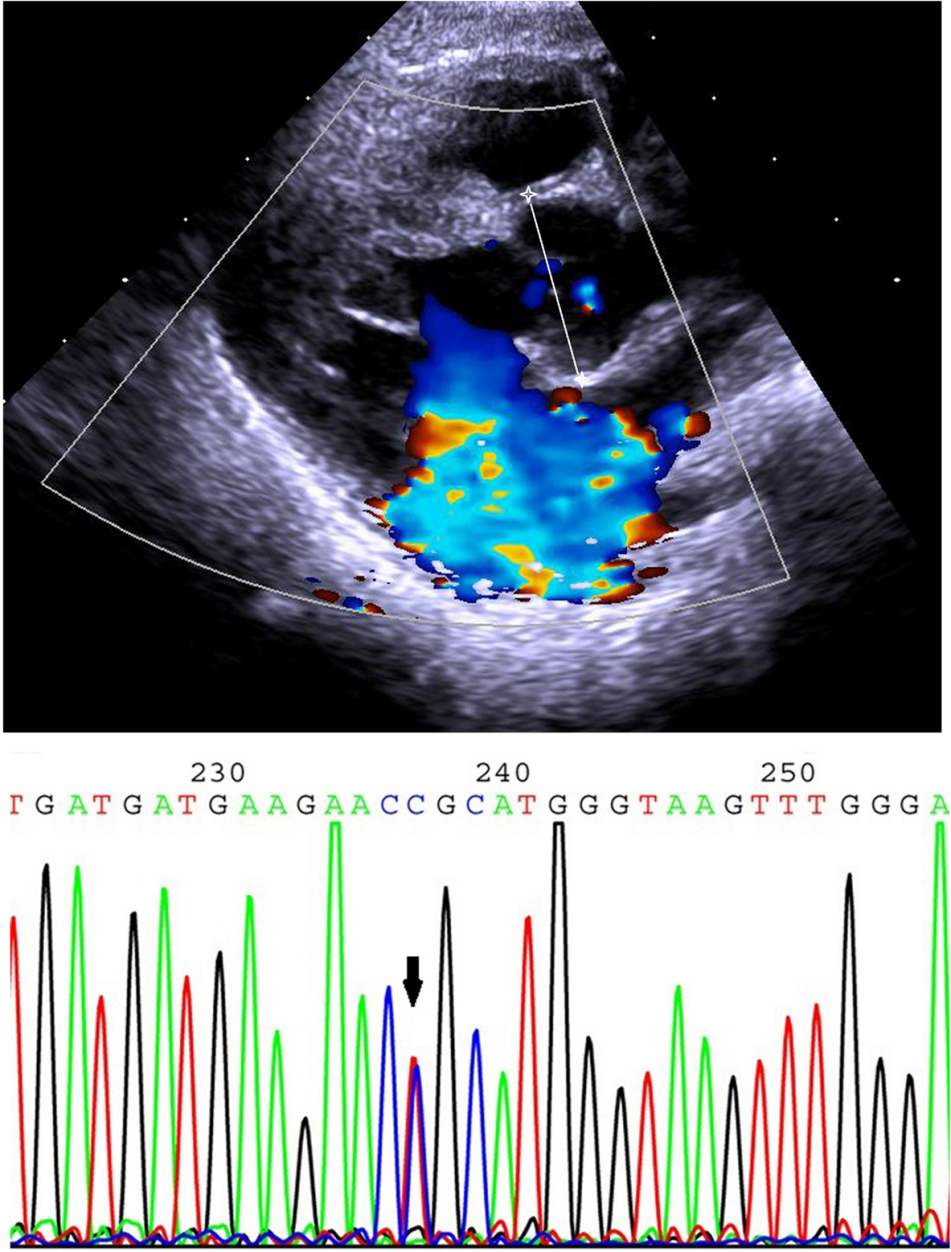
Echocardiograph showing aortic root dilatation at the sinuses of Valsalva (line in-between two asterisks) and the sequencing result showing the heterozygous missense mutation c.3331 T > C in the patient (arrow)
